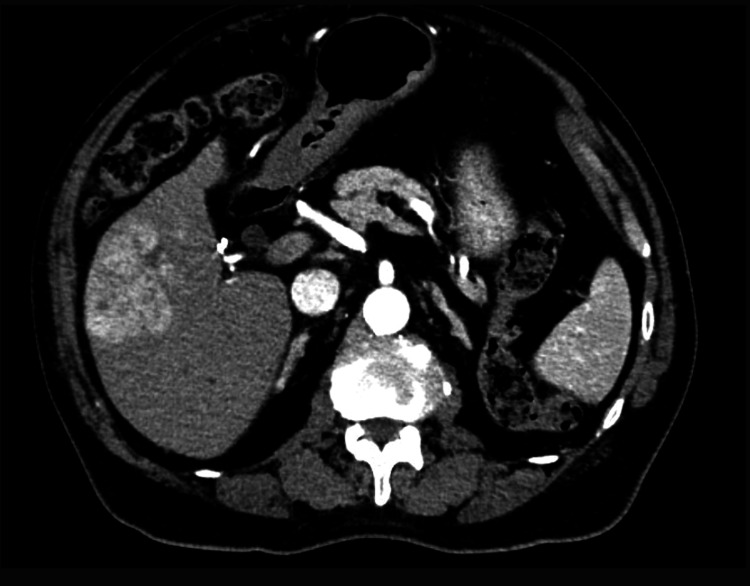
A CT scan of the abdomen showing right liver hepatocellular carcinoma at initial diagnosis
